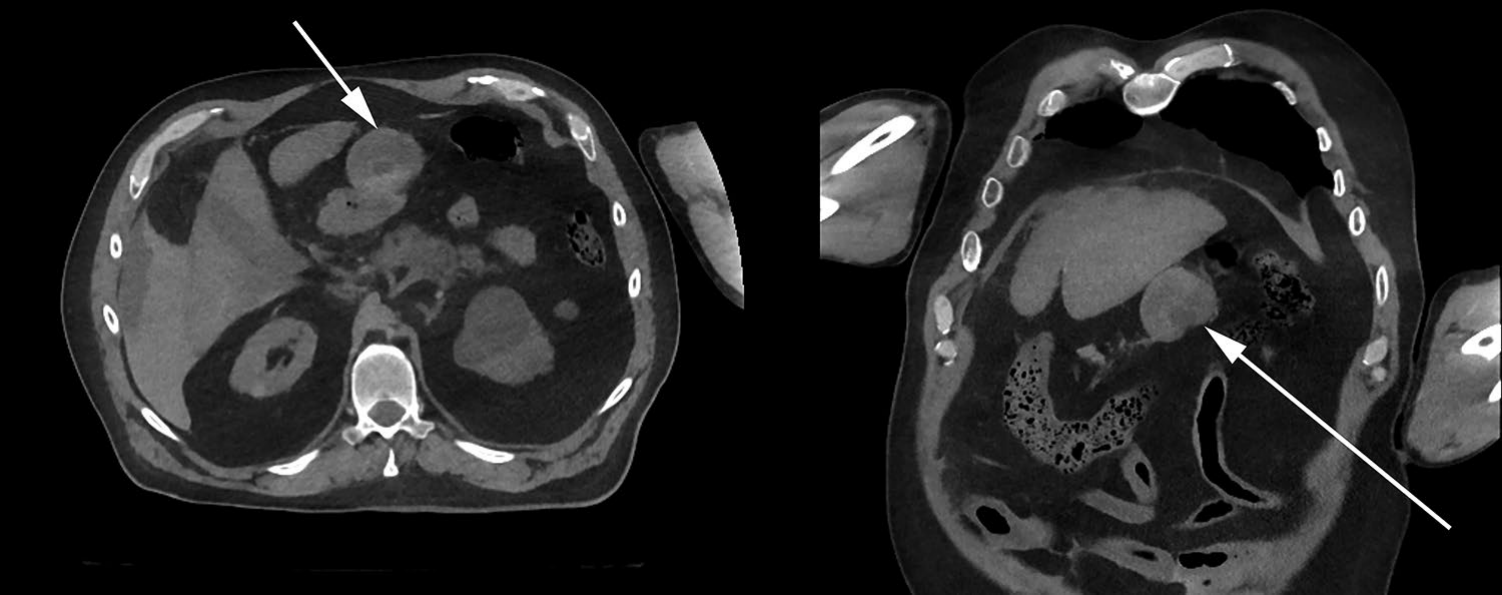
Postmortem CT (left axial slice, right coronial slice) showing visceral aneurysmal lesion (white arrows) and evidence of blood in abdominal cavity
